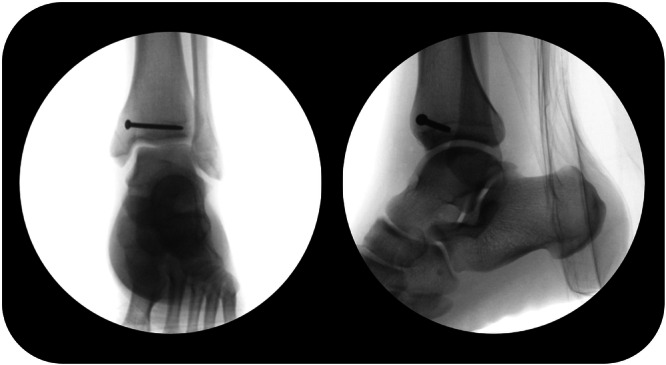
Anterior–posterior and lateral radiograph of the ankle showing screw fixation of the fracture.
